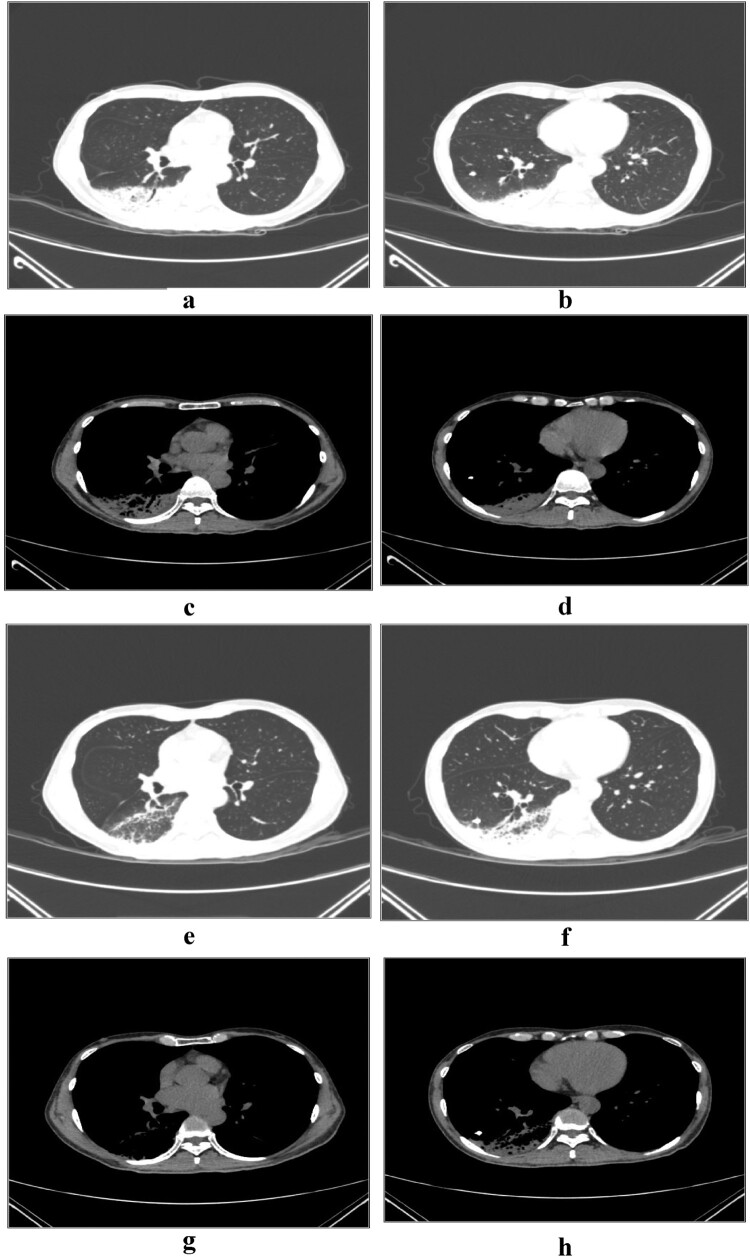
Serial chest computed tomography (CT) scans of the 46-year-old male with psittacosis pneumonia (the third patient). The initial CT scan (3 days after the onset) shows consolidations with bronchograms in the right lower lobe (a, b, c, d). The follow-up CT scan (13 days after the onset) shows the area of consolidation in the right lower lobe has decreased obviously (e, f, g, h).
